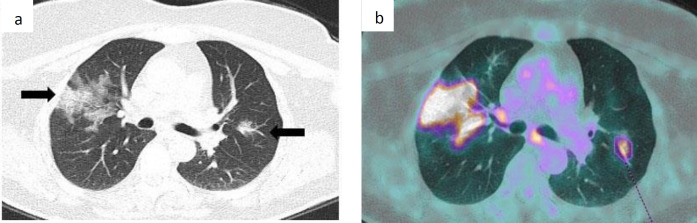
CT (a) and PET-CT (b) images from a 57 years old female in semestral follow-up for a nasopharyngeal cancer and asymptomatic at the moment of the acquisition. In the co-registration CT (a) there are bilateral GGO in the right and left upper lobes (black arrows); no consolidation are noted, resulting in an early stage. These GGO demonstrate intense FDG uptake (b), with SUV_max_ in the right lobe of 11.02 and SUV_max_ in the left lobe of 6, 72. Otherwise, PET was negative
